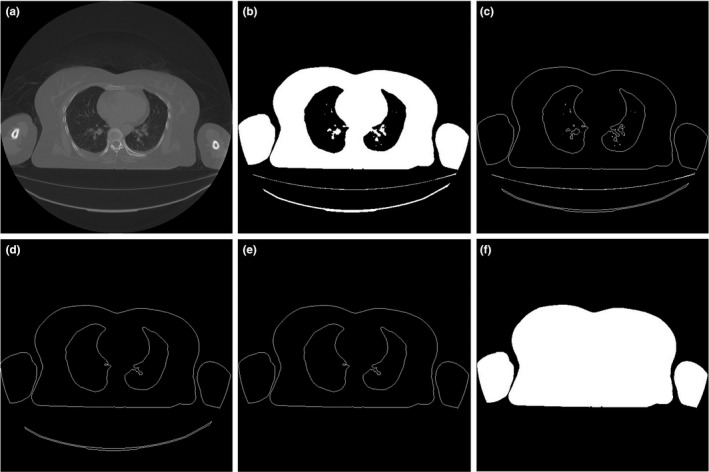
Proposed automated contouring stages: (a) initial image after converting CT data into HU, (b) binary image as a result of thresholding, (c) result of edge detection, (d) image showing the 6 largest objects including the patient table, (e) patient boundary after table removal, and (f) result of automated contouring after assigning all pixels within patient boundary with a value of 1
